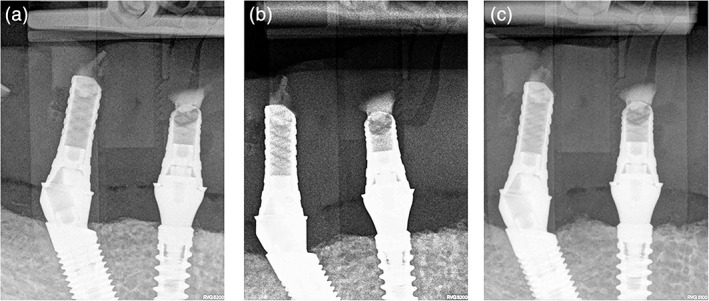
Radiographic image of axial and sloped implants indicating minimal bone loss and survival at 1‐year follow‐up: (a) just after implantation, (b) after 6 months and (c) at 1‐year follow‐up
